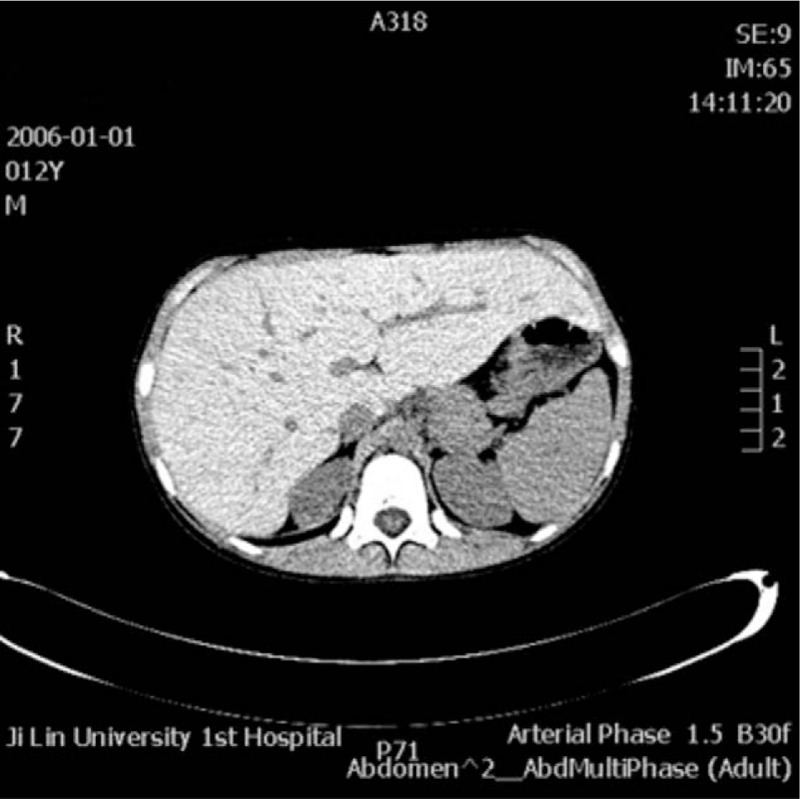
Abdominal noncontrast computed tomography (CT) scan showed marked enlargement of the liver.
